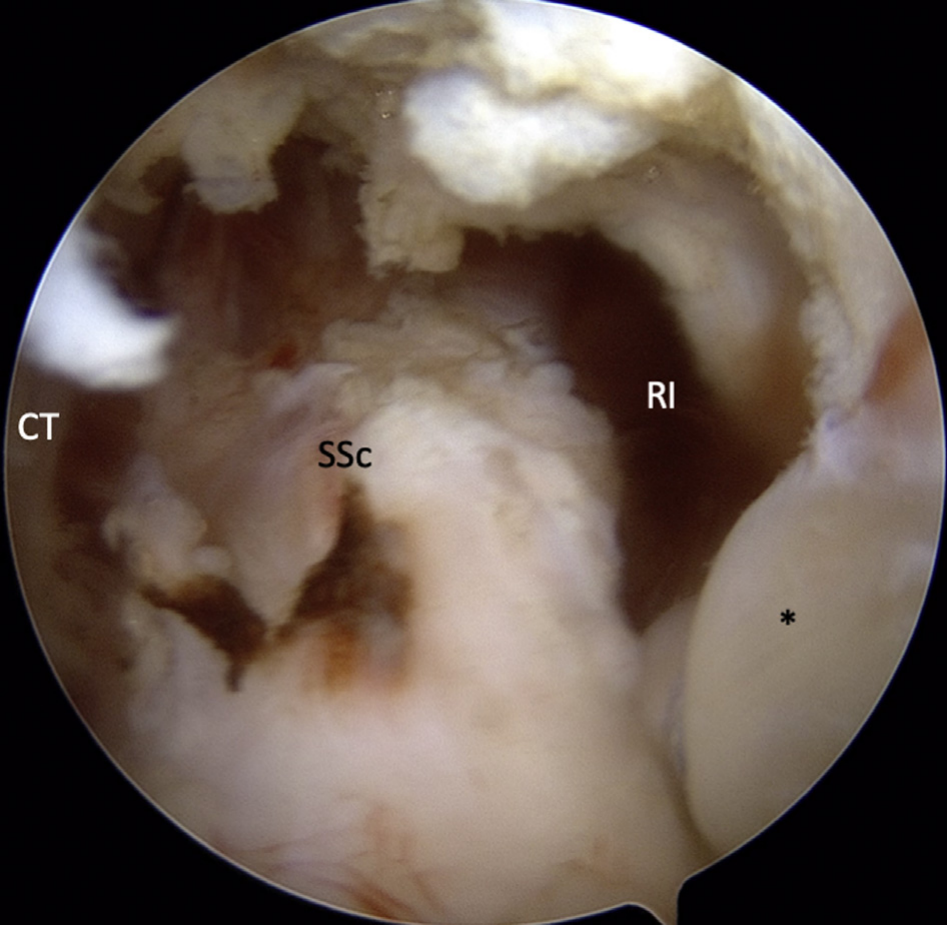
Intra-articular view of a left shoulder, ASL viewing portal. The LHB (∗) passes through the SSc split into the glenohumeral joint and is brought back to anterior portal through the RI to load the interference screw. (ASL, anterosuperolateral; CT, conjoint tendon; LHB, long head of the biceps; RI, rotator interval; SSc, subscapularis.)
